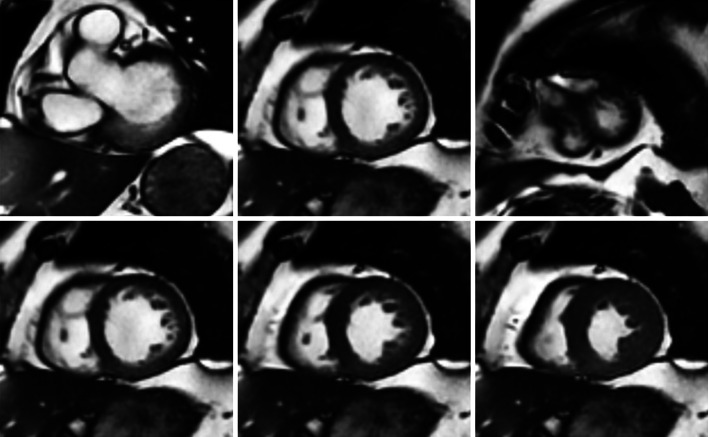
Short-axis cine MR images. *Top row*: slices from base to apex; *bottom row* mid-cavity slice from diastole to systole, displayed using our automatic cardiac segmentation platform GIMIAS. www.gimias.org
